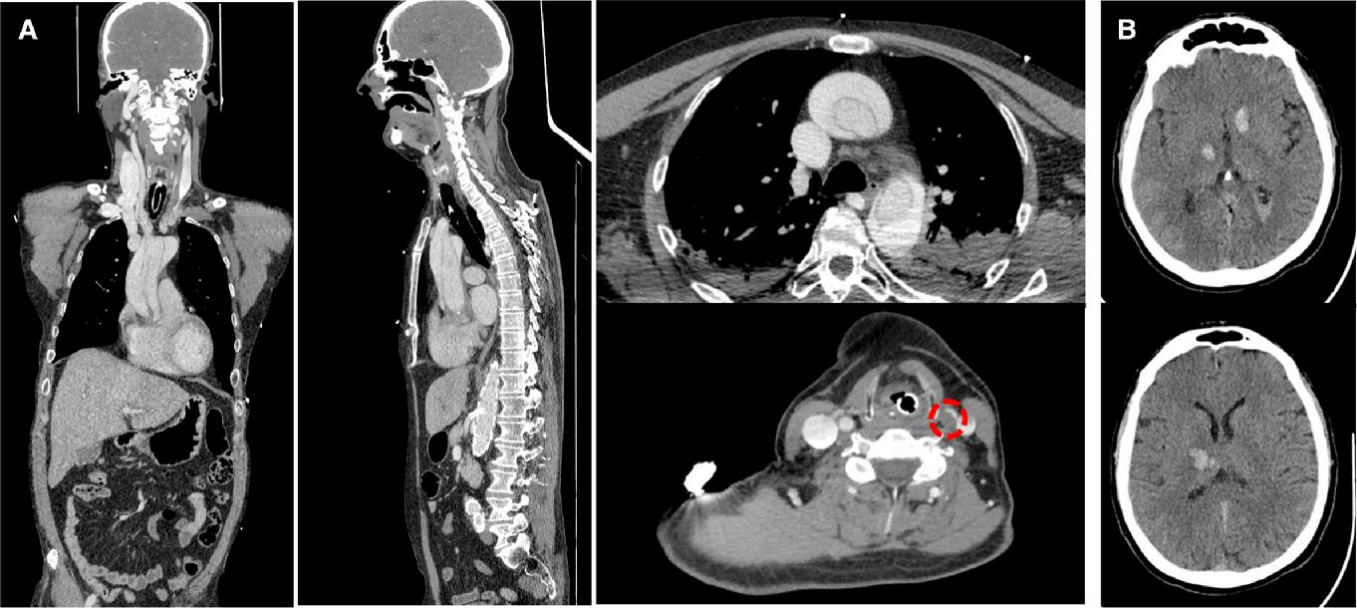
(*A*) Chest and neck computed tomography angiography showing a Stanford Type A acute aortic dissection extending from the ascending aorta to the descending aorta. The false lumen can be seen extending to the left common carotid artery as well (dotted circle). (*B*) Brain computed tomography scan showing two intraparenchymal haemorrhages at the basal ganglia bilaterally and another in between the right internal capsule and the thalamus.
